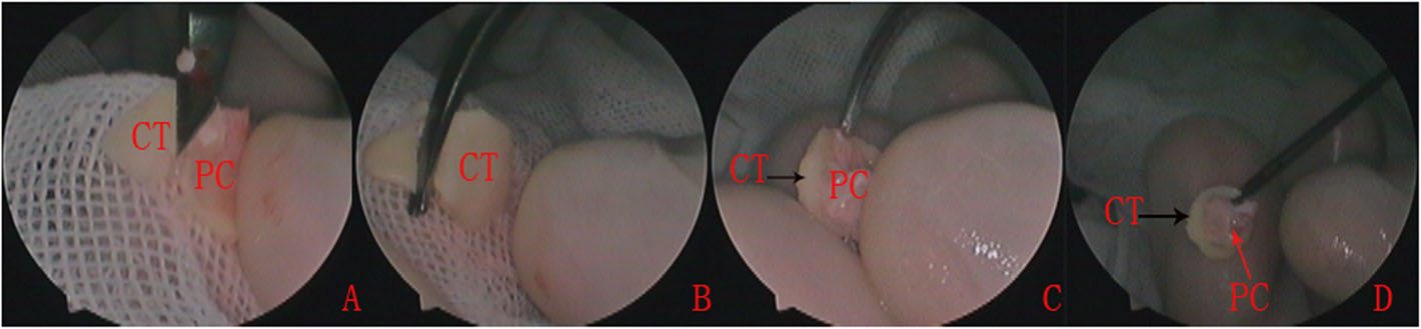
Preparation of a double layer tragal cartilage perichondrium graft. The tragal cartilage with a single layer of perichondrium was harvested (a), the tragal cartilage graft was shaped (b), and the lateral perichondrium was peeled circumferentially and rolled up from the cartilage graft (c and d). PC, perichondrium; CT, cartilage
